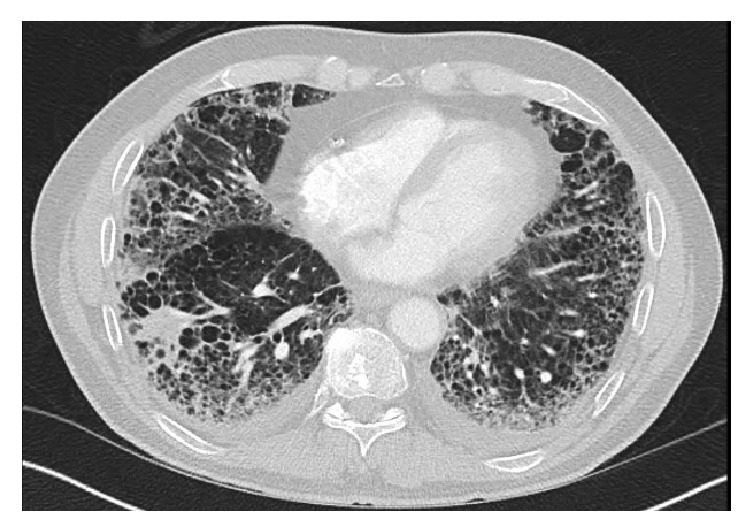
Chest Computed Tomography (CT) angiogram obtained to rule out pulmonary embolism (PE) showed diffuse bilateral pulmonary infiltrates, mediastinal lymphadenopathy, and small bilateral pleural effusions.
